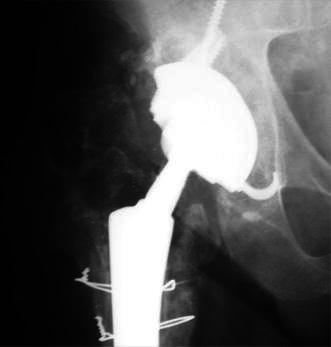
Radiograph after the second operation reveals reimpacted new femoral neck–stem component. Notice the absence of ectopic bone from the lesser trochanter area
